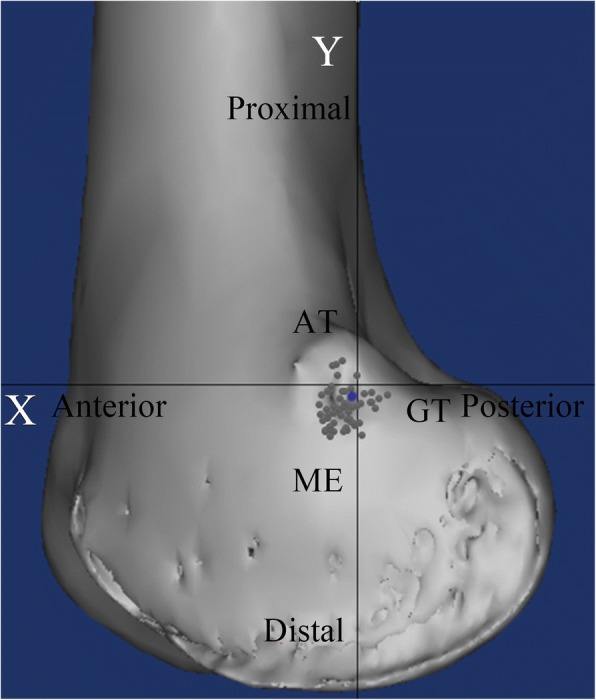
A representative computed tomography image showing the distribution of the locations of all the medial patellofemoral ligament femoral tunnels. The blue circle indicates the location of Schöttle point, the black circle indicates the centers of the medial patellofemoral ligament femoral tunnels in all 60 reconstructed knees. AT: adductor tubercle; ME: medial epicondyle; GT: gastrocnemius tubercle
